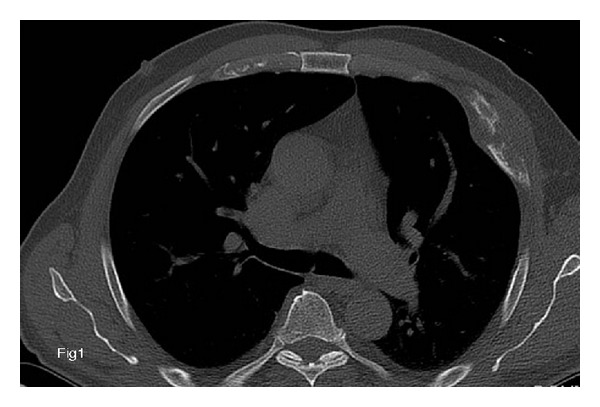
Axial CT scan for 65-year-old man with papillary thyroid microcarcinoma: large lytic mass of the anterior arch of the left 4th rib with infiltration of the soft tissues.
